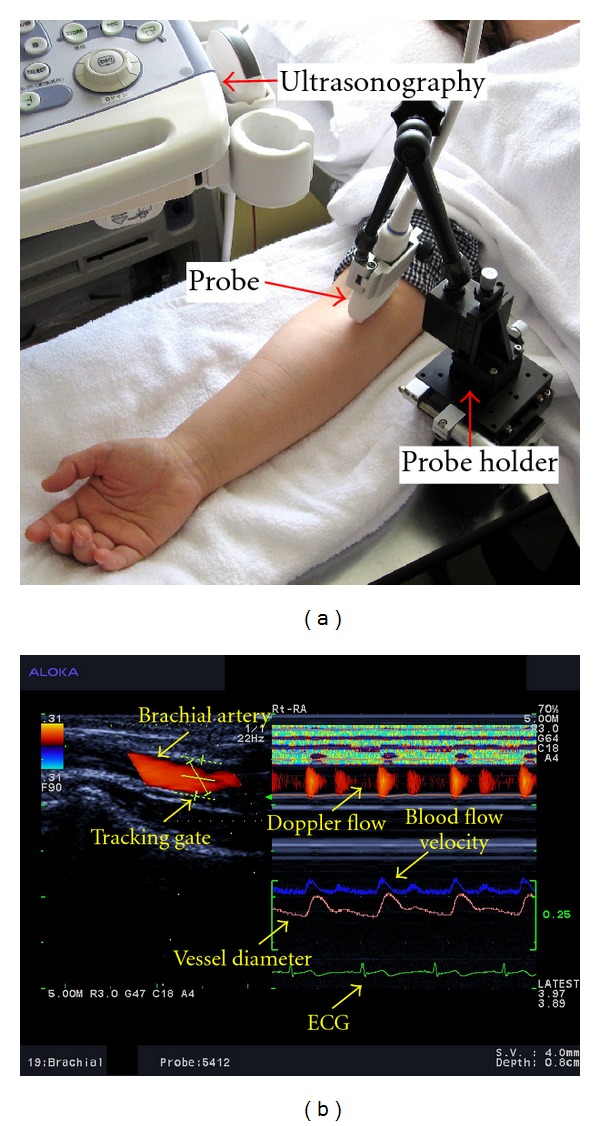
(a) An ultrasonographic measurement of the brachial artery with a special probe holder (MP-PH0001®, Hitachi-Aloka Medical Ltd., Tokyo, Japan). (b) Hemodynamic data obtained by ultrasonography. The left image shows the vessel image and position of the tracking gate of the brachial artery. The right image shows the changes in vessel diameter, Doppler flow, and blood flow velocity determined by an automated edge detection device and computer analysis software (e-tracking system®, Hitachi-Aloka Medical Ltd., Tokyo, Japan).
